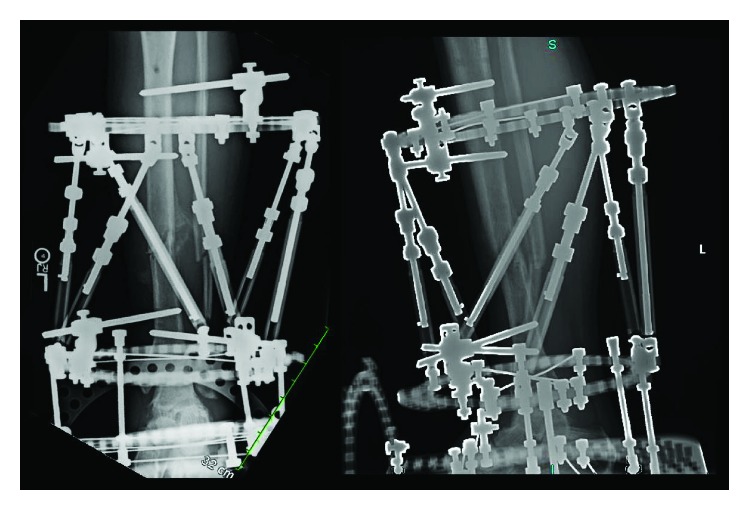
AP and lateral radiographs of the left leg demonstrating deformity correction after a 27-day period.
